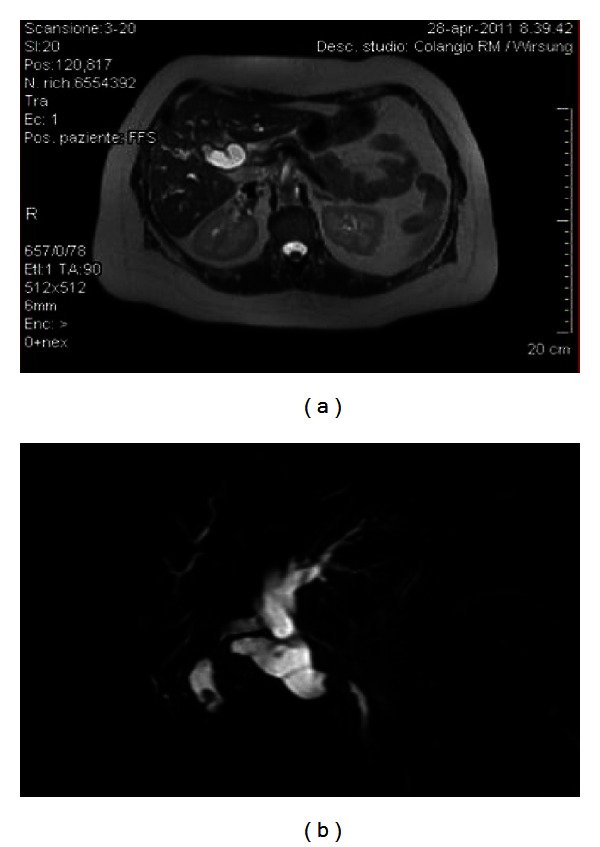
(a) MRI showing a sclero-atrophic cholecystitis with an endoluminal stone. (b) Cholangiographic reconstruction showed an absence of signal in the pre-papillary tract of CBD, with intrahepatic duct dilatation of the left lobe of the liver.
